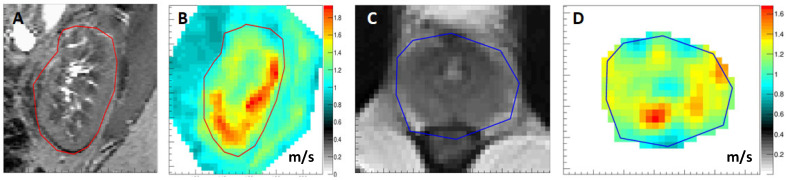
Three-dimensional MRE results in the kidney and the prostate. The outlines of the kidney and the prostate are highlighted in red and blue respectively. (A,B) T2-weighted anatomical image of the kidney and corresponding image of the shear wave speed [m/s]. Data from University Hospital Vienna, Austria. (C,D) T2-weighted anatomical image of the prostate and corresponding image of the shear speed [m/s]. Data from University Hospital Frankfurt am Main, Hesse, Germany. The institutional ethical review board approved this prospective study. Written informed consent was obtained from the participants.
